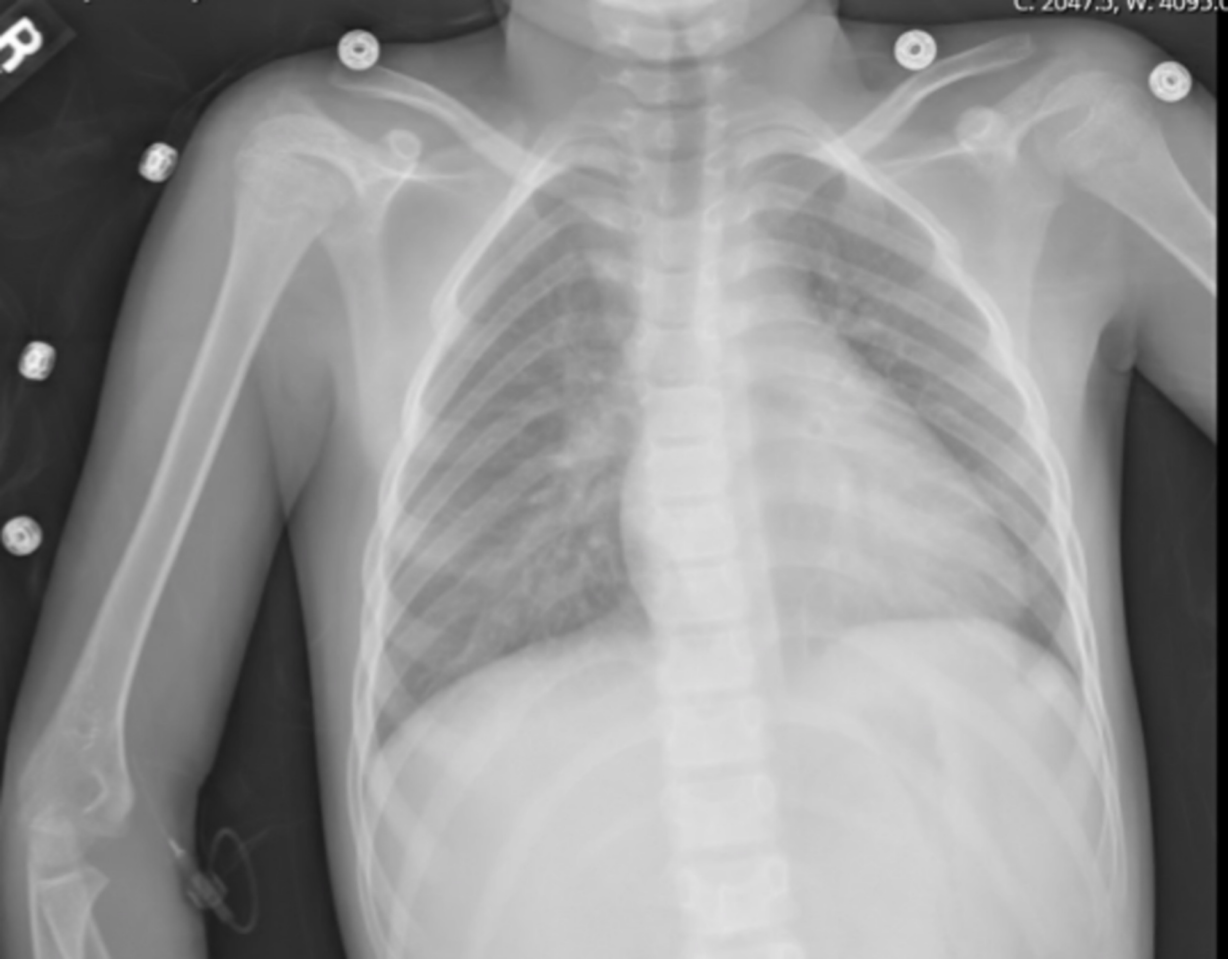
Chest x-ray showing perihilar peribronchial thickening without focal consolidation
